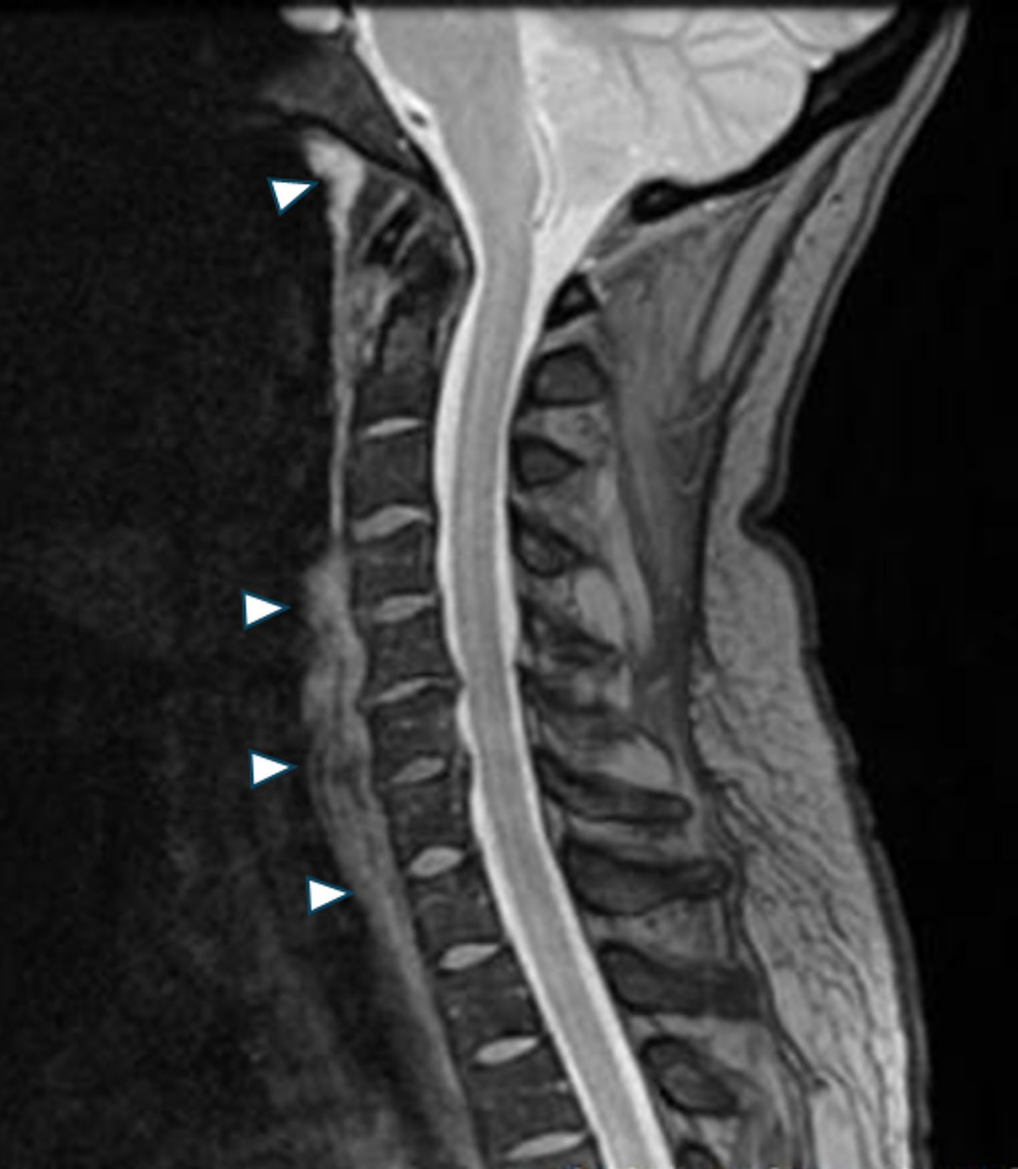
Sagittal T2 MRI of a patient with *Longus Colli Calcific Tendinitis*, it is not possible to clearly identify a calcific deposit on the anteroinferior border of the anterior arch of C1. It is notable the presence of a hyperintense image along the anterior aspect of the cervical spine running from C1 to T3 in the retropharyngeal space, corresponding with soft tissue edema (white arrow heads)
